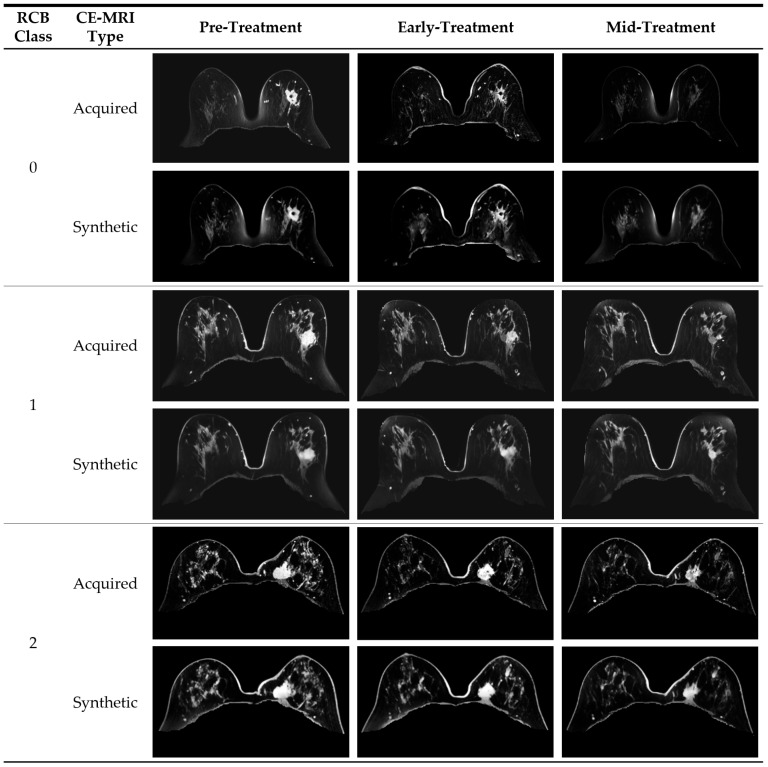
Example axial-view acquired vs. synthetic CE-MRI at pre-treatment, early treatment, and mid-treatment for study patients with treatment outcomes of RCB class 0, 1, and 2. Images shown are post-contrast fat-suppressed T1-weighted images (acquired) and corresponding synthesized CE-MRI representations, where the tumors are each bright at pre-treatment, and exhibit varying degrees of enhancement at early and mid-treatment. Each pair of images (acquired and synthetic) are shown at the same spatial location through the central portion of the tumor. Top: 53-year-old woman with a Grade 3 invasive ductal carcinoma, ER−/PR−/HER2+, Ki-67 not available, with RCB class 0 outcome to NAT. Middle: 63-year-old woman with a Grade 3 invasive ductal carcinoma, ER−/PR−/HER2−, Ki-67 50% with RCB class 1 outcome to NAT. Bottom: 49-year-old woman with a Grade 1 invasive ductal carcinoma, with ER+/PR+/HER2−, Ki-67 15%, with RCB class 2 outcome to NAT.
